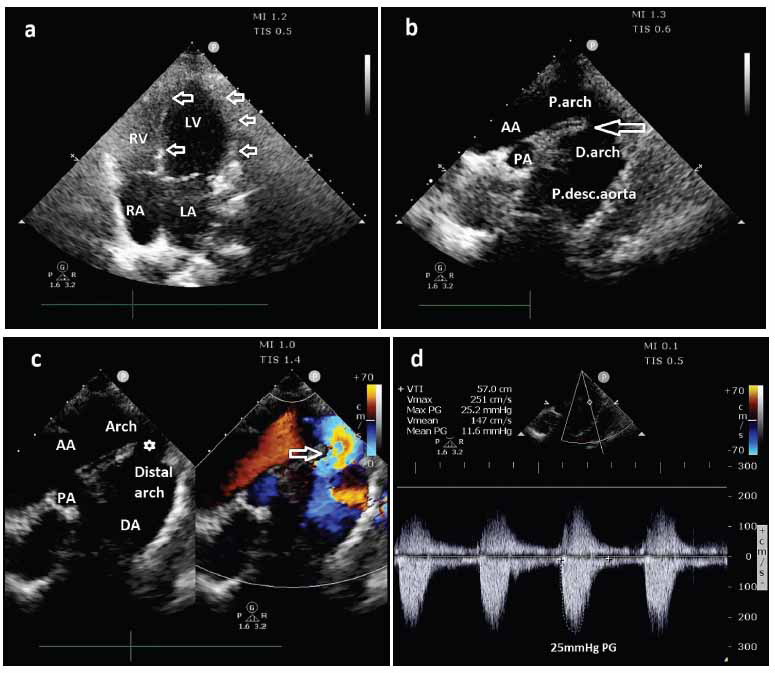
2-D ECHO images in (a) apical four-chamber (A4C) view showing normal chambers (LV-left ventricle, RV-right ventricle, LA-left atrium, and RA-right atrium) and absence of left ventricular hypertrophy (white hollow arrows), (b) Suprasternal view showing features of coarctation of the arch of the aorta with mild dilatation of the descending aortic segment (AA-ascending aorta, PA-pulmonary artery, P.arch-proximal arch, D.arch-distal arch, p.desc.aorta-proximal portion of the descending aorta) (c) suprasternal view showing colour doppler across the lesion demonstrating mild turbulence (AA-ascending aorta, DA-descending aorta, PA-pulmonary artery, white hollow star-shows 'coarcted' segment, white hollow arrow shows mild turbulence across the aortic arch), and (d) in the suprasternal view continuous wave doppler showing a gradient of 25mmHg.
